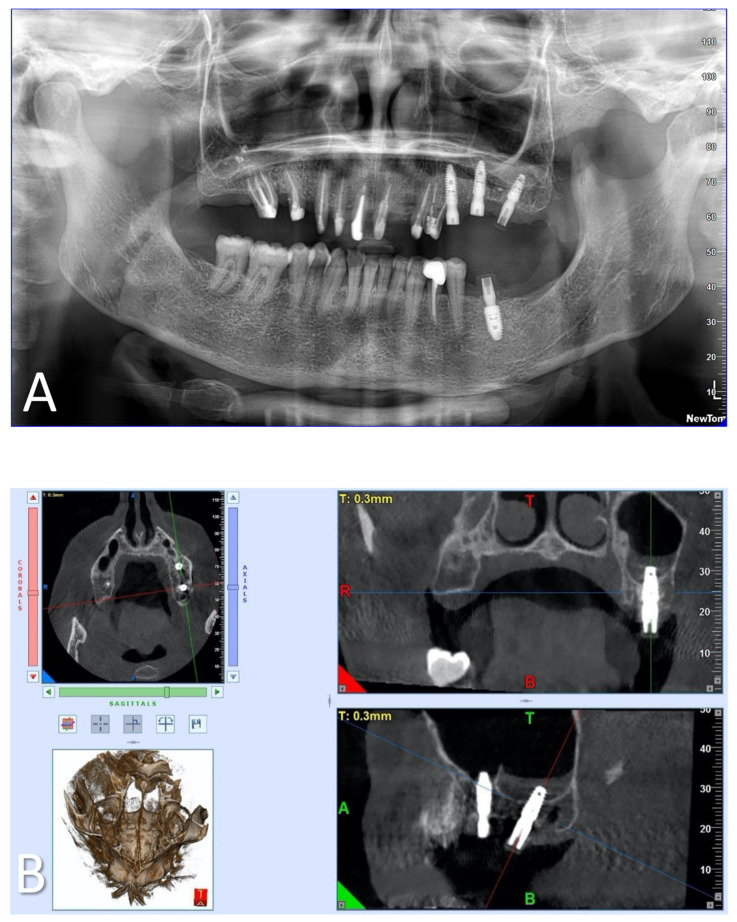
(A) OPT study after 45 days of healing and (B) cone–beam study after 45 days of healing: blue: axial planes; red: coronal planes; green: sagittal planes; T: top; B: bottom; R: right; L: left; A: anterior; P: posterior.
